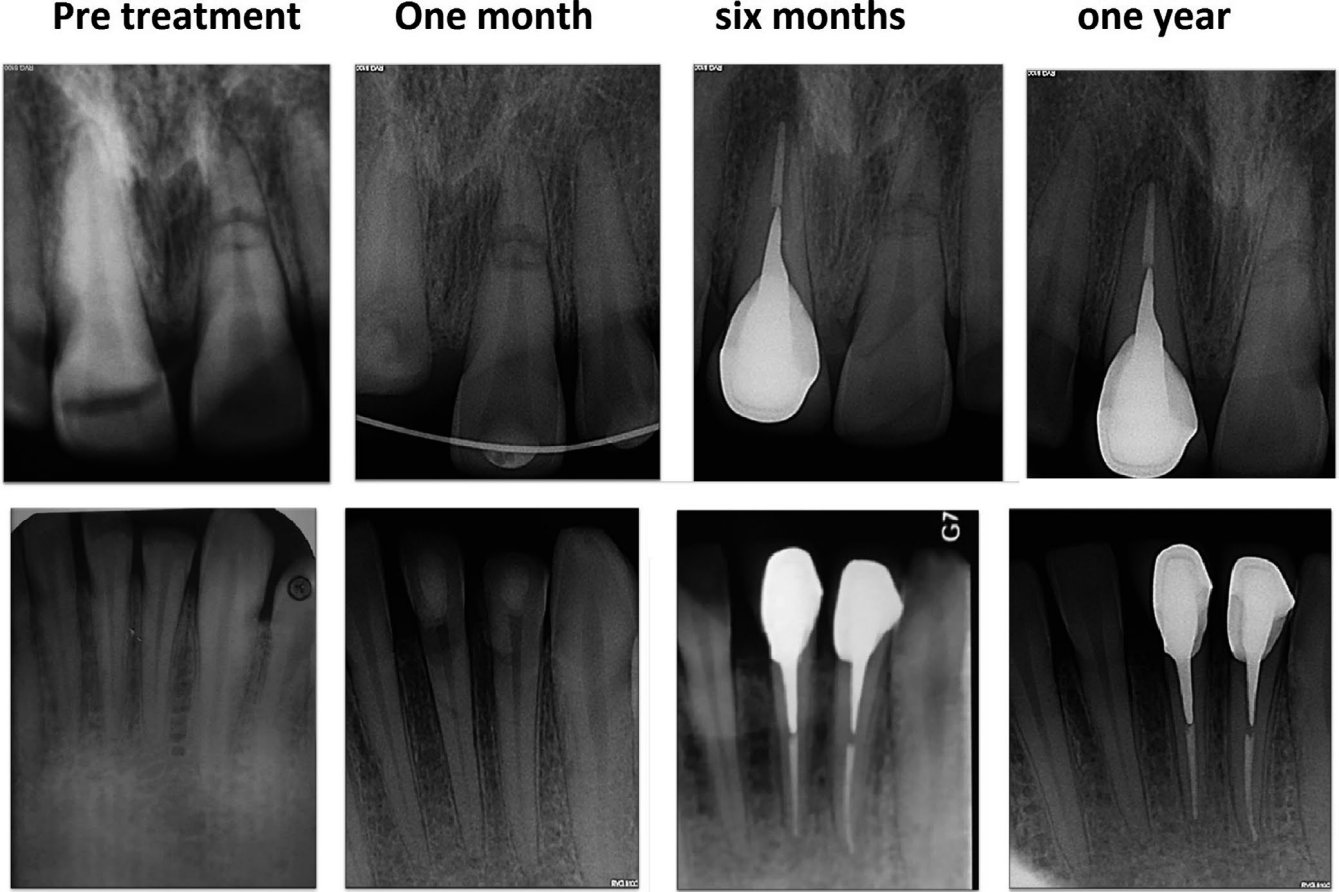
Follow‐up radiographs for teeth #8 and #9 (top) and teeth #23 and #24 (bottom)
